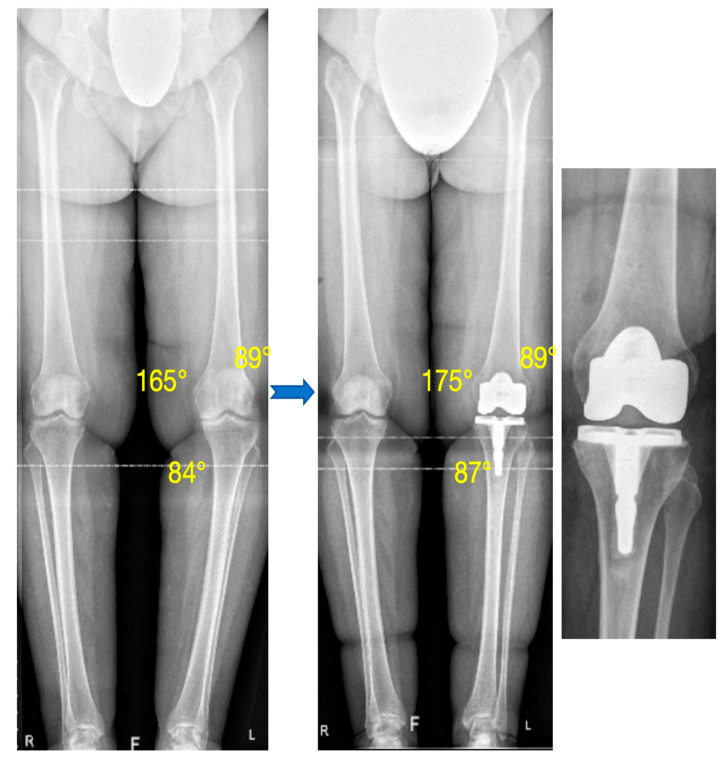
Preoperative and postoperative standing long-leg radiograph. The preoperative varus (preoperatively, HKA: 165°, mMDFA: 89°, MPTA: 84°) was partially corrected with the TKA with restoration of the femur and partial correction of the tibial varus (postoperatively, HKA: 175°, mMDFA: 89°, MPTA: 87°).
